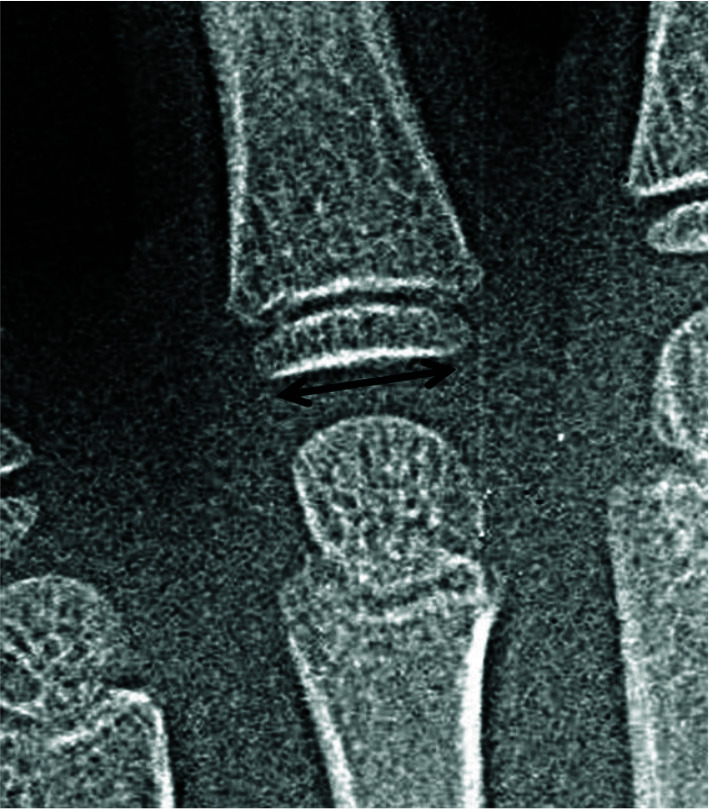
4^th^ metacarpophalangeal joint thickness in hand radiograph
